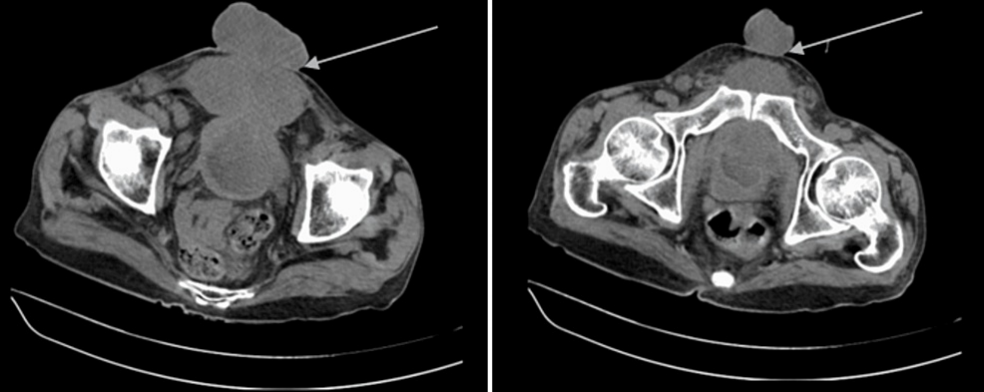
Non-contrast computed tomography scan showing large exophytic and endophytic components of polypoidal lobulated soft tissue mass lesion originating from the wall of urinary bladder and also mild cortical irregularity and erosions in superior pubic ramus and pubic symphysis (left and right).
